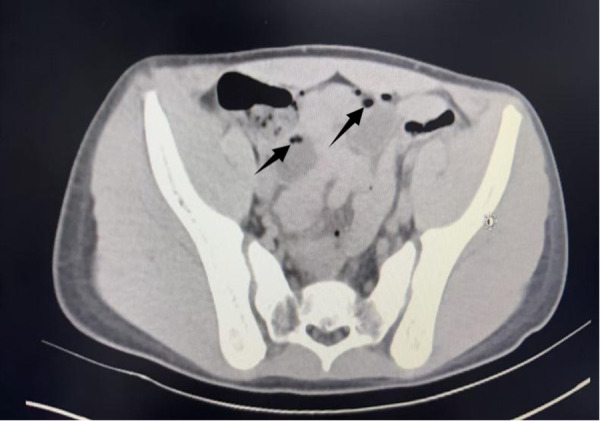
Preoperative pelvic CT showing free intraperitoneal air.
